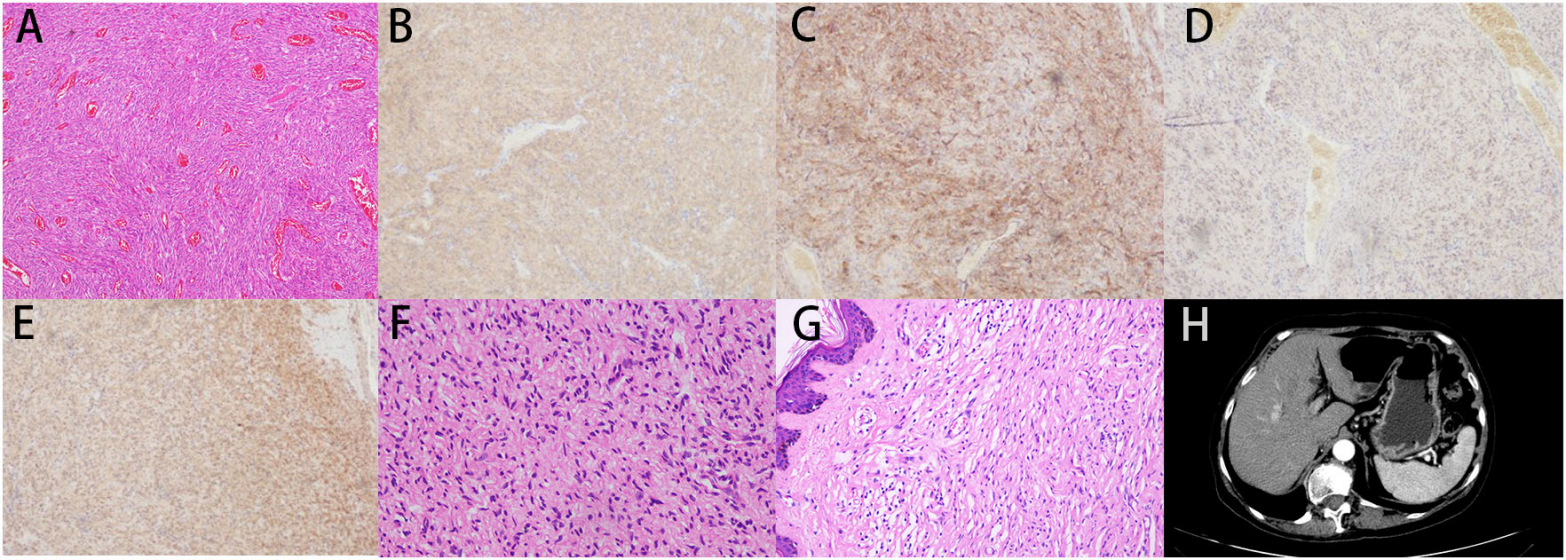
(A) Pathological section of intestinal mass at 100x magnification, showing numerous spindle-shaped cells. (B) Intestinal mass: *CD117*(+). (C) Intestinal mass: *CD34*(+). (D) Intestinal mass: *SDHB*(+). (E) Intestinal mass: *vimentin*(+). (F) Patient 1: Postoperative pathological section of skin mass, 200x. (G) Pathological section of the daughter’s skin tumor, 200x. (H) Three months later, a follow-up CT scan was performed, and the anastomosis was restored to normal. No liver metastasis was found.
